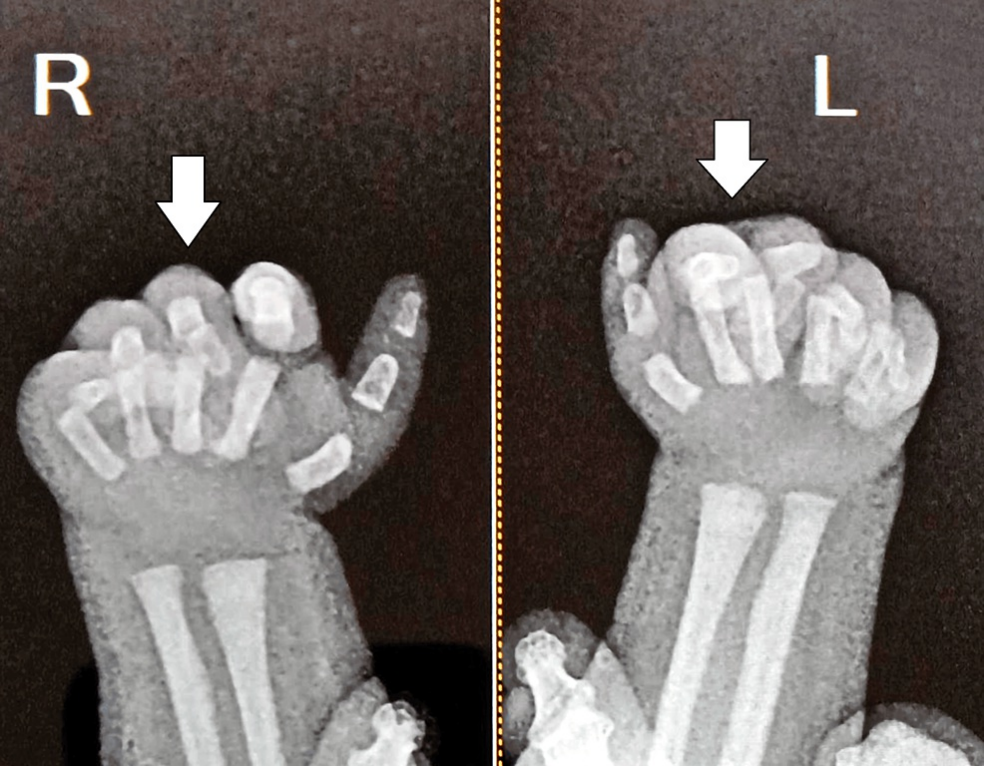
The X-ray of both hands and wrists showed features consistent with AMC, including camptodactyly and radial deviation of the fingers (arrows)
